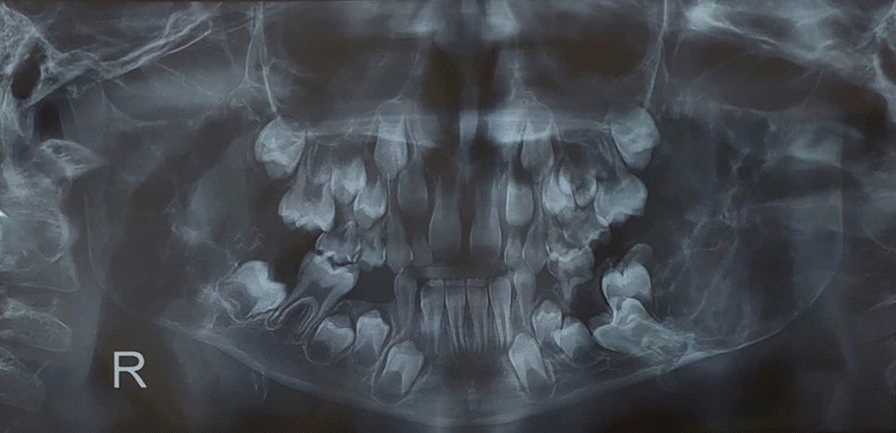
Panoramic radiograph from case 8 demonstrating bilateral multilocular radiolucent lesions involving the posterior mandible, with thinning and expansion of cortical plates and displacement of developing teeth, consistent with cherubism
